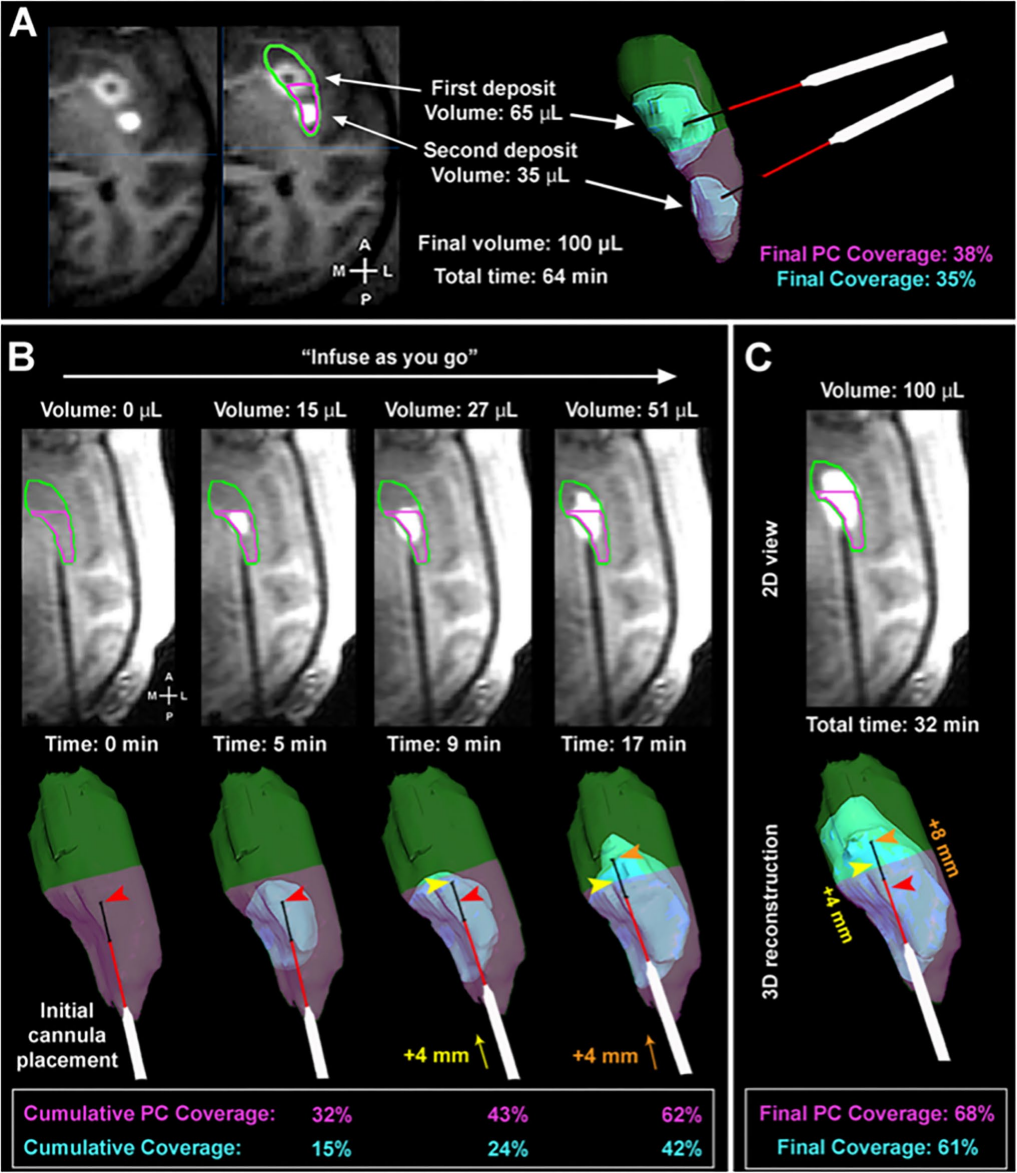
Case depiction and artist’s rendition (A) of a convective infusion via stereotactically placed cannula. Infusion of drug mixed with Gd-DTPA allows for tracking of distribution. (B-E) Real-time, serially obtained coronal MR images with hypodense tumor region in the pons (arrows), showing growth of the perfused area, with total tumor coverage obtained in (E). Figure adapted from Lonser et al. 2007 [16]
